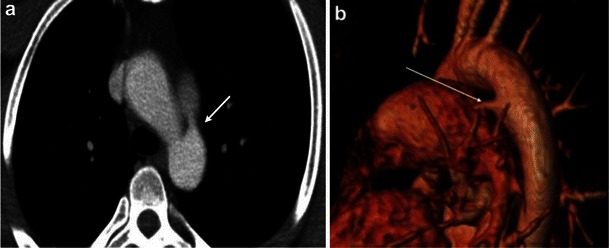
Contrast-enhanced axial CT image (a) and a volume rendered image (b) in a patient with patent ductus arteriosus (*PDA*) depicting the persistent communication between the pulmonary artery and descending aorta (*arrow*). The flow direction in the post-natal period is aorta to pulmonary artery as the pulmonary pressures decrease. This can lead to pulmonary hypertension, which on CT will present as enlarged pulmonary trunk as seen on the volume rendered image
